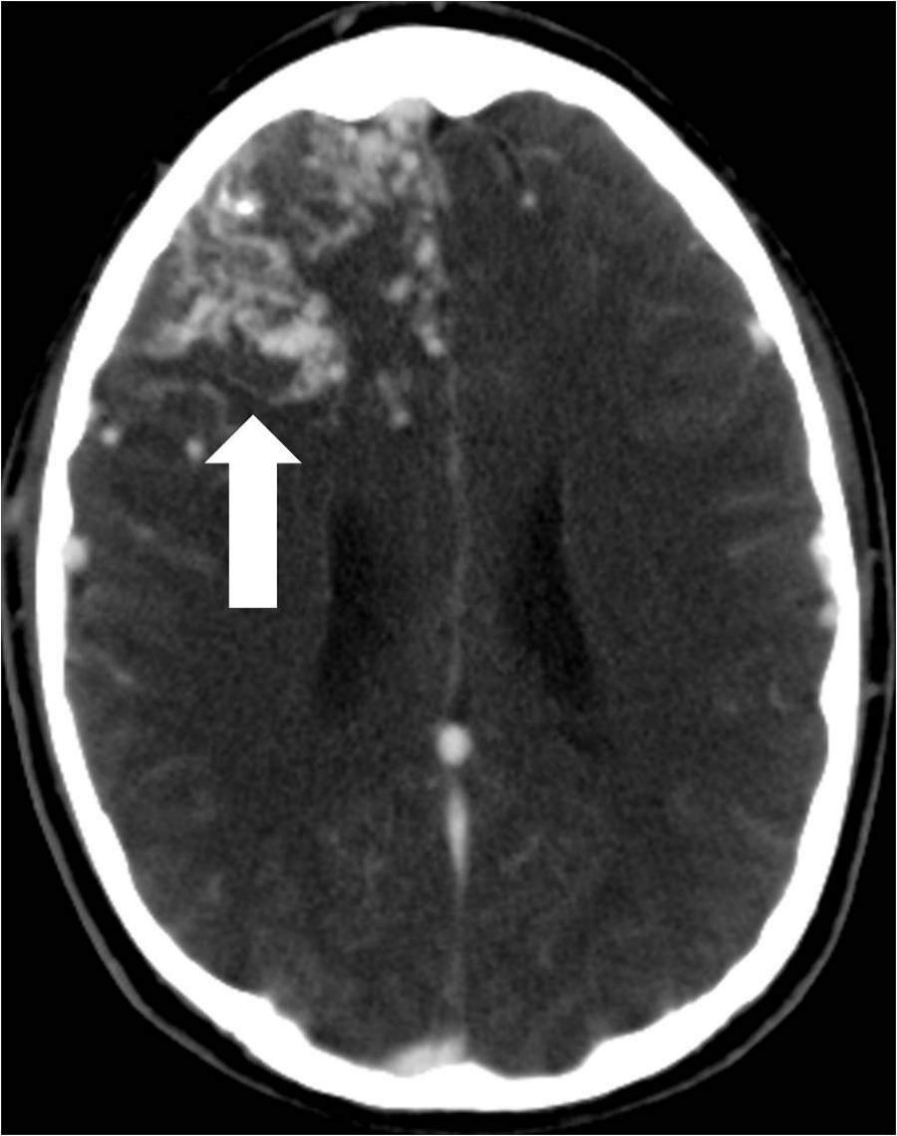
Computed tomography angiogram of the head shows the large enhancing right frontal lobe arteriovenous malformation nidus (arrow).
